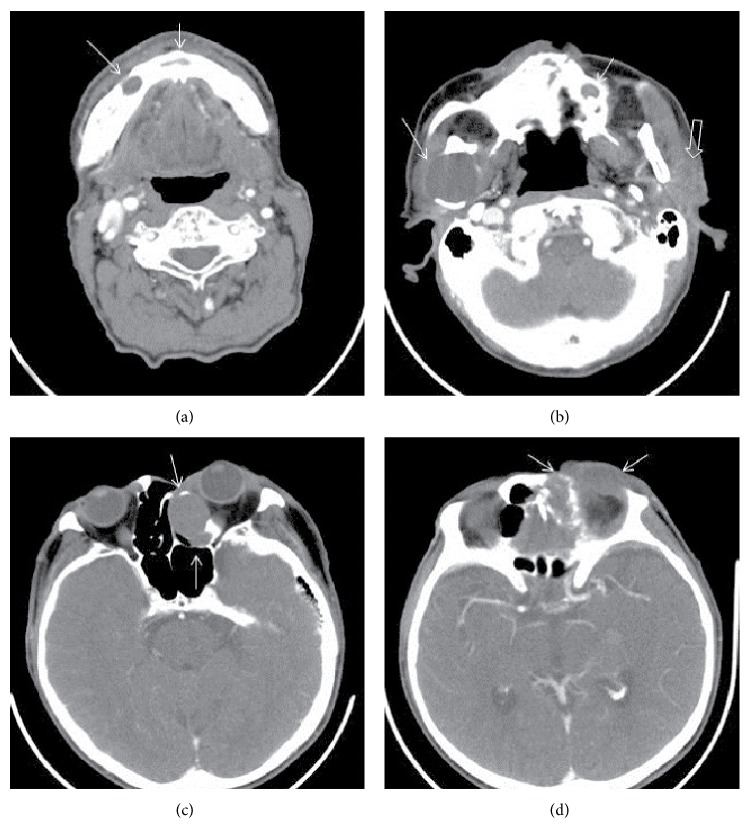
Contrast enhanced CT images of neck: (a) cystic mass lesions with regular margins at mandibular corpus (arrow marked). (b) A round cystic lesion with lytic expansil regular contour at right ramus mandible and small cystic lesion at maxillar bone (marked arrow), a heterogeneous contrasted mass at left parotid region (light arrow marked). (c) Two lytic expansil cystic lesions at left ethmoidal cells. (d) A heterogeneous contrasted mass with irregular margins at subcutaneus tissue of left orbital wall with destruction of frontal bone.
